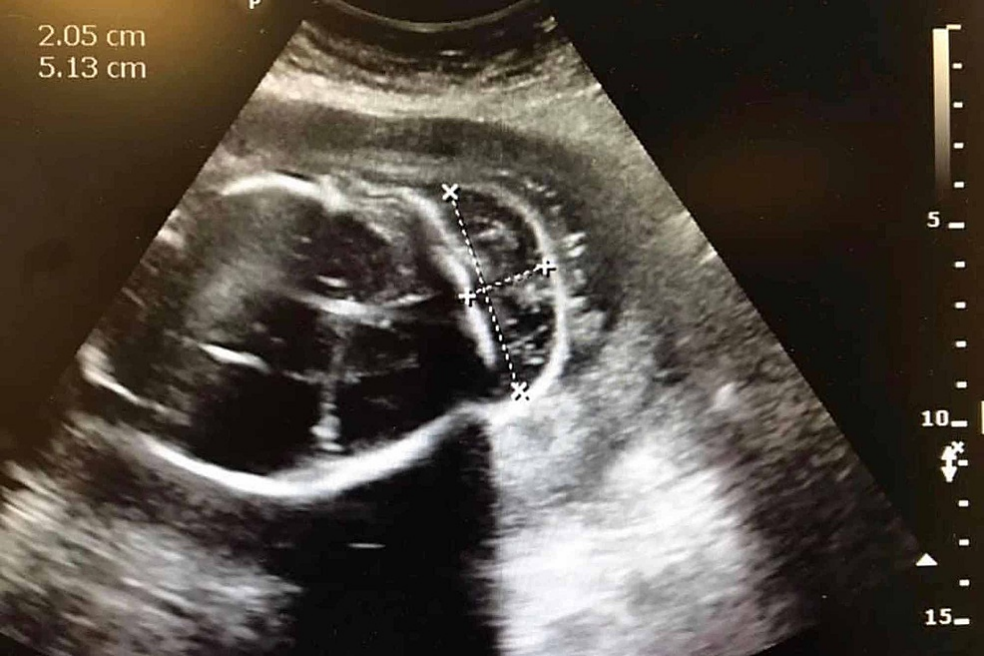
Fetal ultrasound, axial view. The dimensions indicate the size of the lesion at 2 cm by 5 cm. Posterior aspect of the skull is depicted on the right side of the image.
